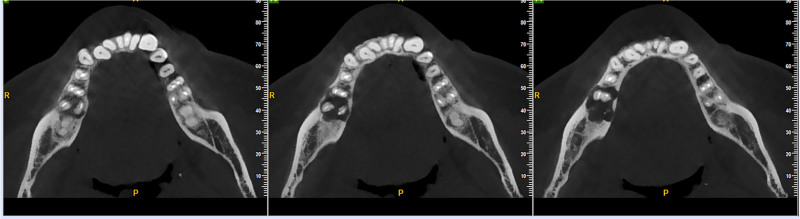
CBCT horizontal view: a patchy irregular low-density lesion in the periapical areas of the right mandibular second premolar and the first molar. The density inside the cyst is uniform, the boundary is clear, and it has a shallow lobulated appearance. The buccal bone plate is thinned due to expansive compression, and there is a small cyst-like erosion and destruction in the local lingual bone plate, with a slight external root resorption. CBCT = cone-beam computed tomography.
